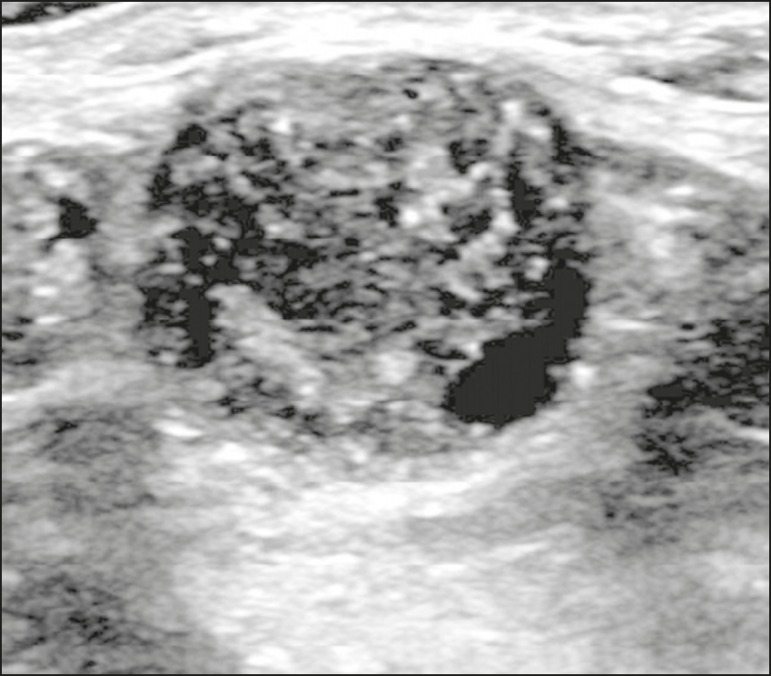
Ultrasound showing a rounded nodule with circumscribed, hypoechoic
